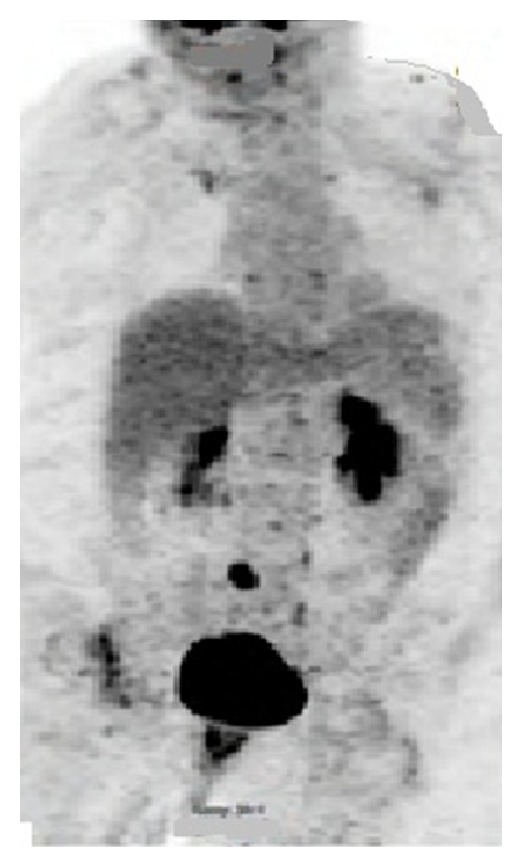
PET CT scan with avid FDG uptake associated with the sigmoid colon.
